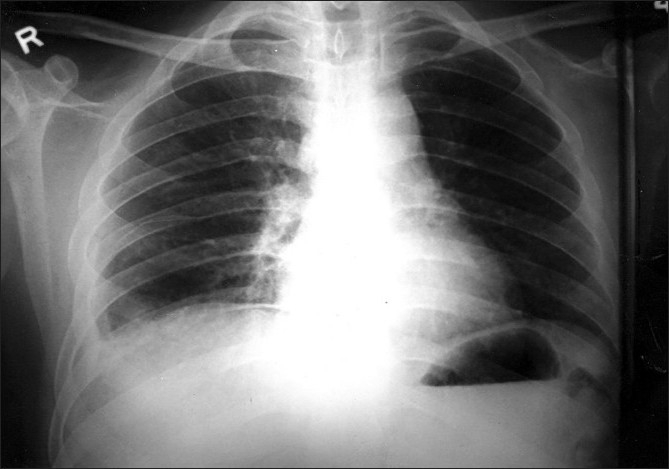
Post whole lung lavage chest radiograph, showing normal study
